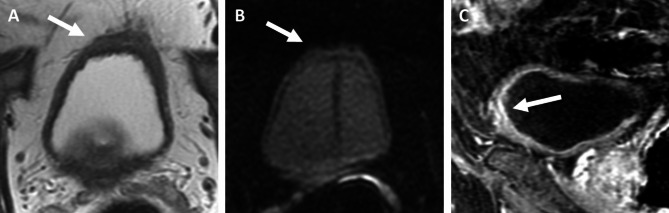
Seventy-two-year old man with high grade muscle-invasive urothelial bladder cancer on transurethral resection. MRI performed after 3 cycles of neoadjuvant gemcitabine + cisplatin, showed residual focal wall thickening (arrow) in the anterior bladder wall on axial T2-weighted imaging (A). There was no abnormal high signal on axial diffusion-weighed imaging (B) but mild ill-defined enhancement was noted on sagittal post-contrast MRI (C). At radical cystectomy, there was a 0.5-cm focus of residual tumor in situ (ypTis). Patient was alive without recurrence at 792 days after surgery
